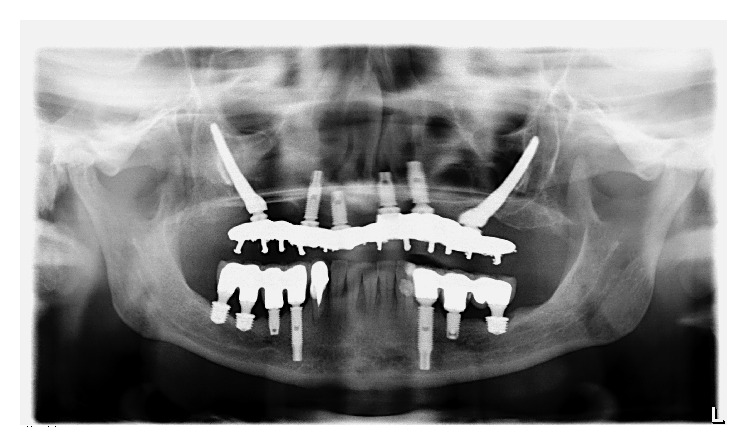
Panoramic radiograph at 2 years of follow-up.
